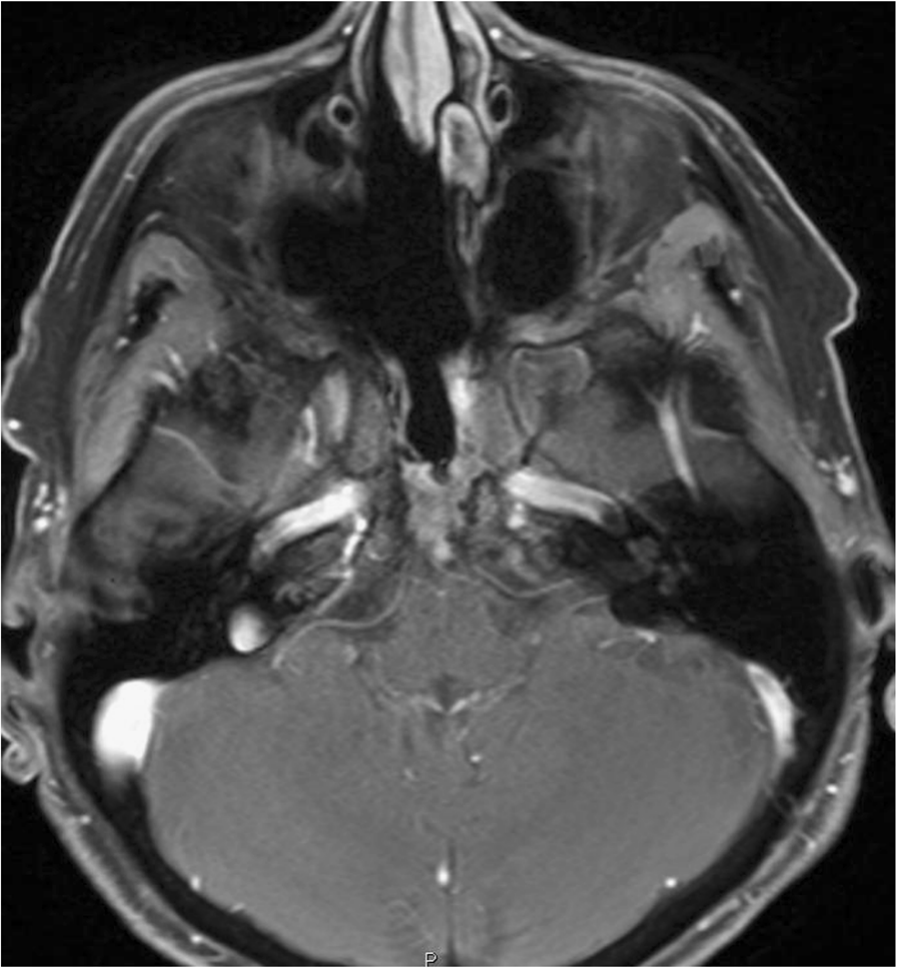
T1-weighted fat suppressed images show no evidence of residual or recurrent tumor.
